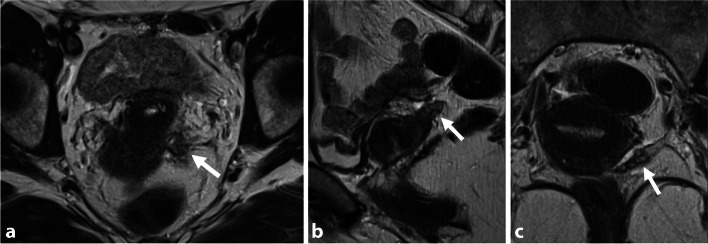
Left utero-sacral ligament (USL) varicosity in a 46-year-old woman with chronic catamenial pelvic pain. a Axial, (b) sagittal, and (c) coronal T2-W MR images show a thickened and pseudonodular left USL (arrows) due to tubular and serpiginous T2-hyperintense veinous structures. No pelvic endometriosis was found at surgery; a pelvic venous congestion syndrome was then suggested
